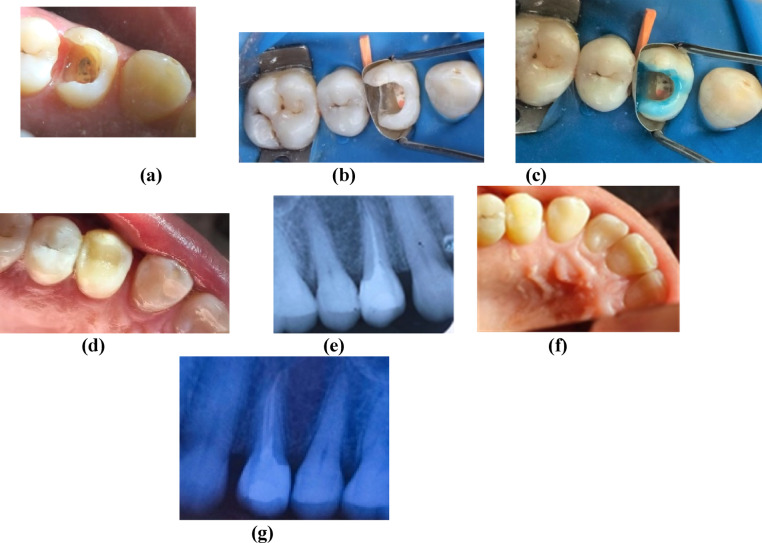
Steps of preparation, restoration, and 3 months of follow-up. (a) Cavity preparation, (b) rubber dam isolation, matrix, and wedge placement, (c) restorative procedure, (d) after final restoration, (e) radiograph after final restoration, (f) clinical photo after 3 months of follow-up g-radiograph after 3 months of follow-up.
